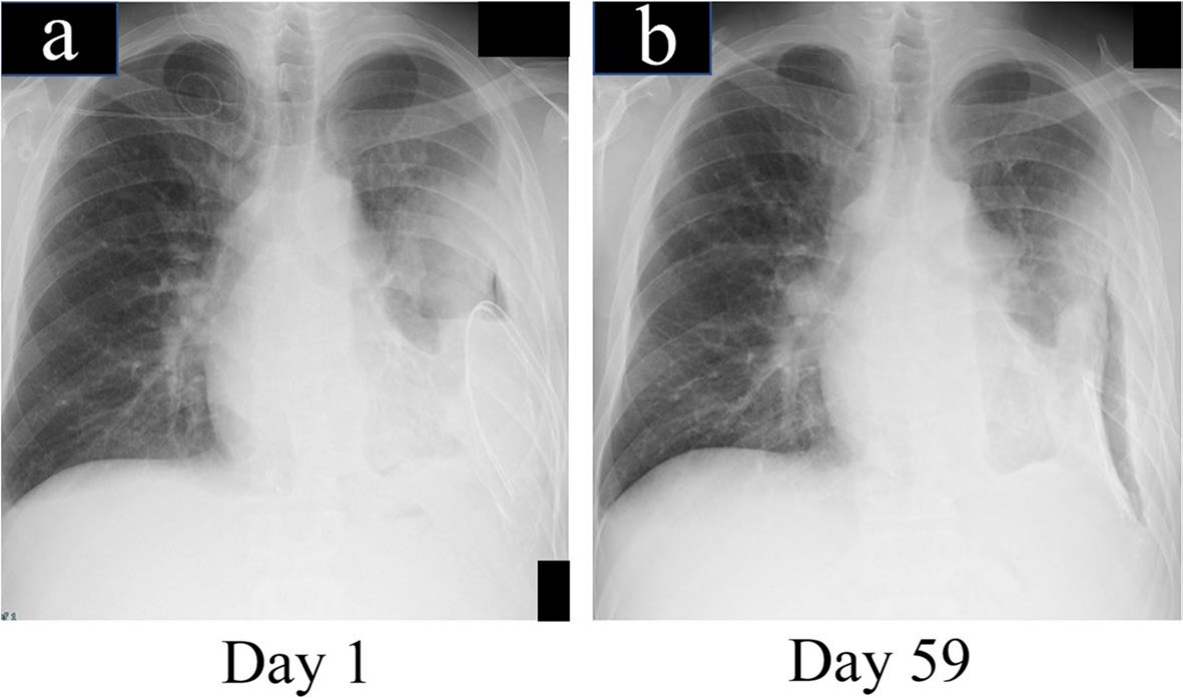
Postoperative X-ray findings after the first surgery. a Chest X-ray on the first day after the first surgery. b Chest X-ray with negative-pressure wound therapy, 59 days after the 1st surgery
